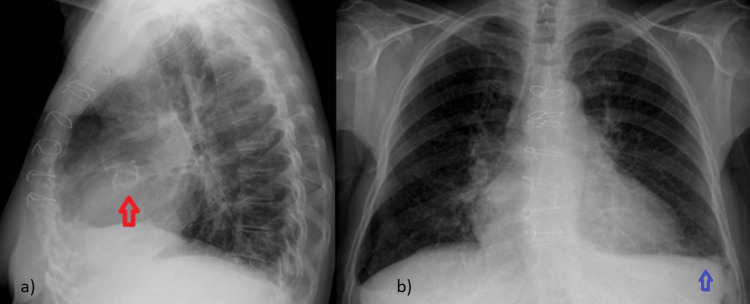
Plain chest radiograph images. (a) Lateral and (b) posteroanterior images reveal blunting of the left costophrenic angle (blue arrow) and the silhouette of the aortic valve replacement (red arrow). Additionally, cardiomegaly is observed.
